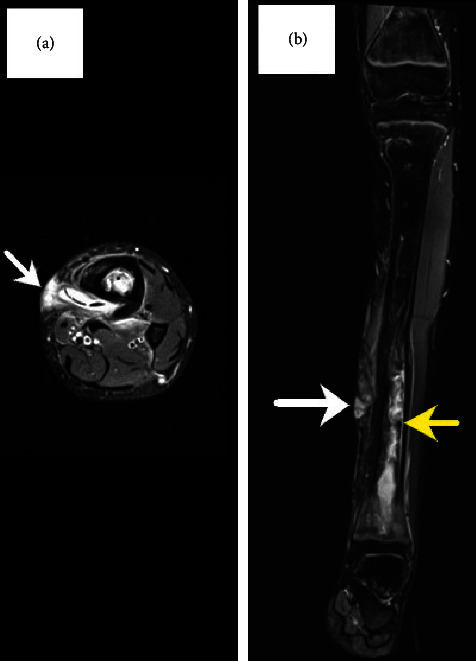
Magnetic resonance imaging (MRI) with gadolinium enhancement of the left leg on (a) transverse view and (b) coronal view demonstrating intramedullary edema (yellow arrow) and the sinus tract (white arrow).
